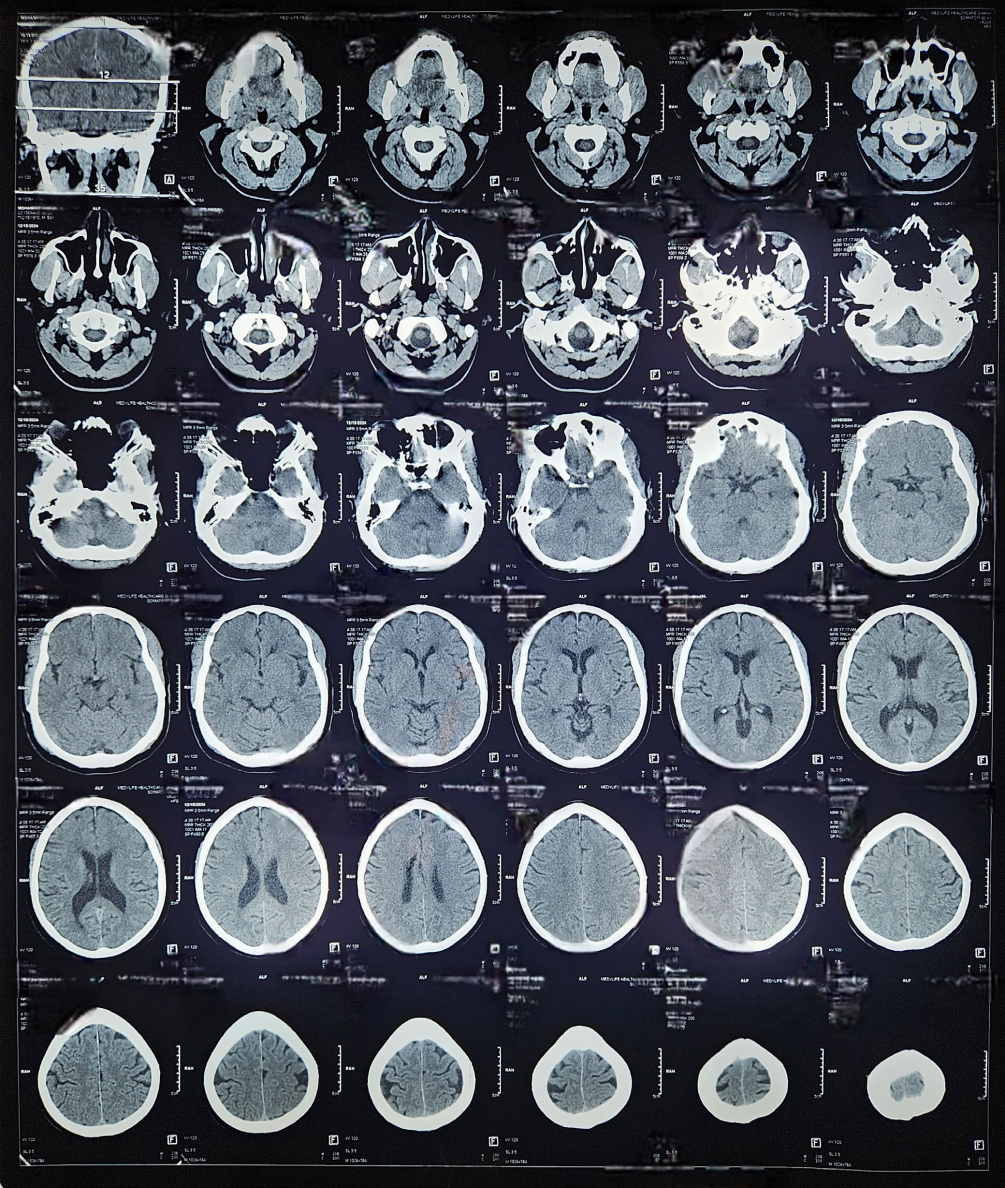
Noncontrast head CT scan showing no abnormalities.
